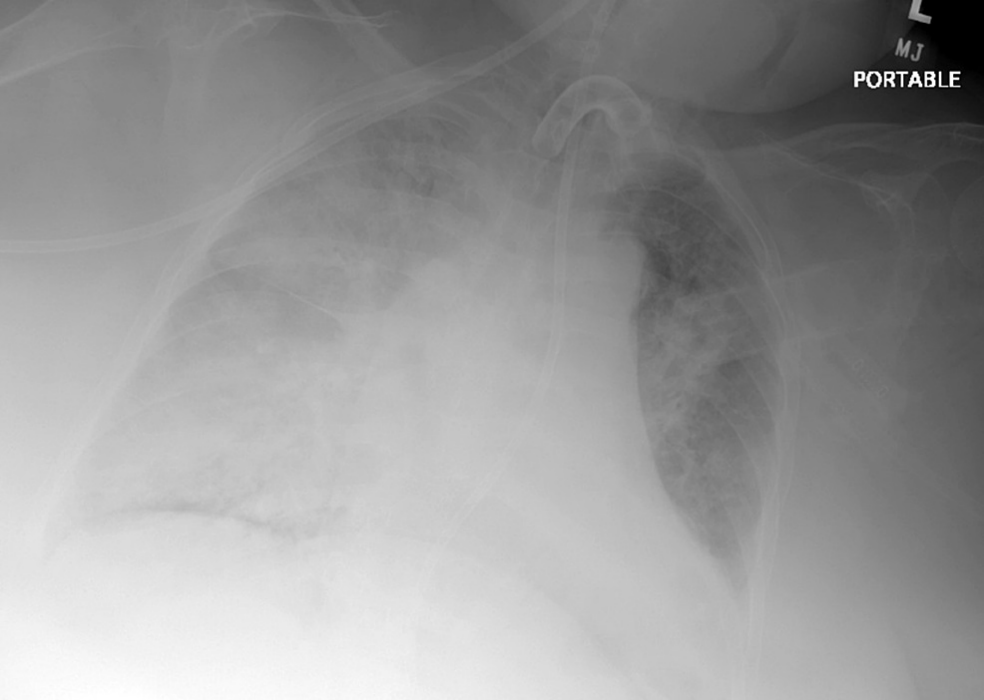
Chest X-ray obtained during the patient's episode of respiratory distress revealing increased/worsening right-sided pulmonary consolidation/edema
